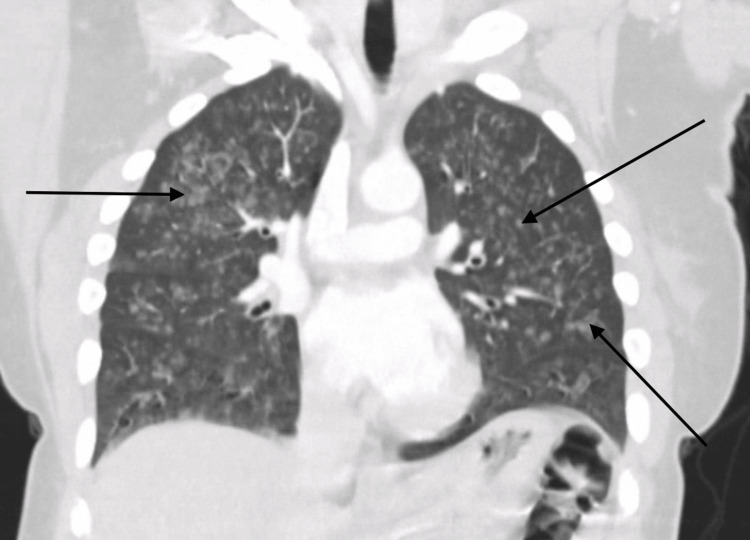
Computed tomography of the chest. Black arrows indicating diffuse ground-glass opacities.
